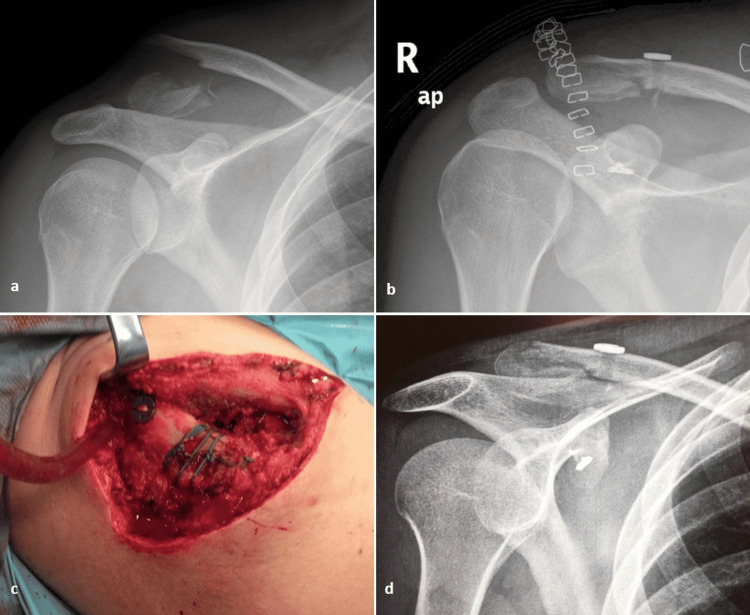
Radiological evaluation and intraoperative photo of patient 2. (a) Preoperative radiograph of an extralateral distal clavicle fracture, with comminution and marked vertical displacement. (b, c) Postoperative radiograph and intraoperative photo showing reduction of the fracture and additional interfragmentary suture repair in respect. (d) Final follow-up radiograph at six months showing maintenance of reduction.
